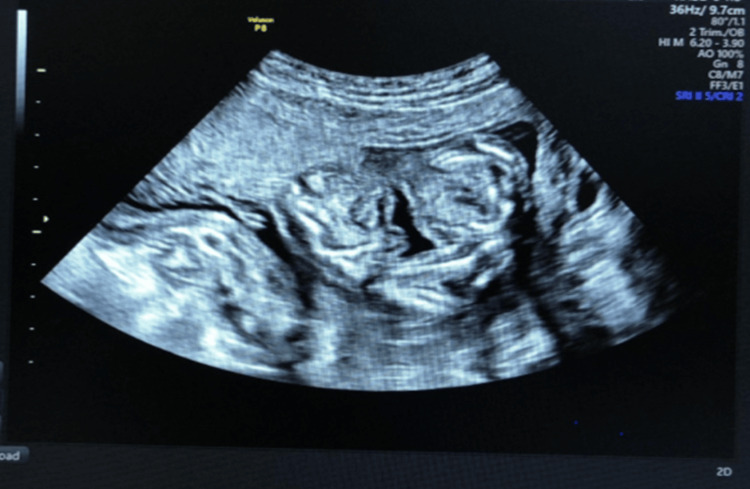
Two-dimensional ultrasound image
